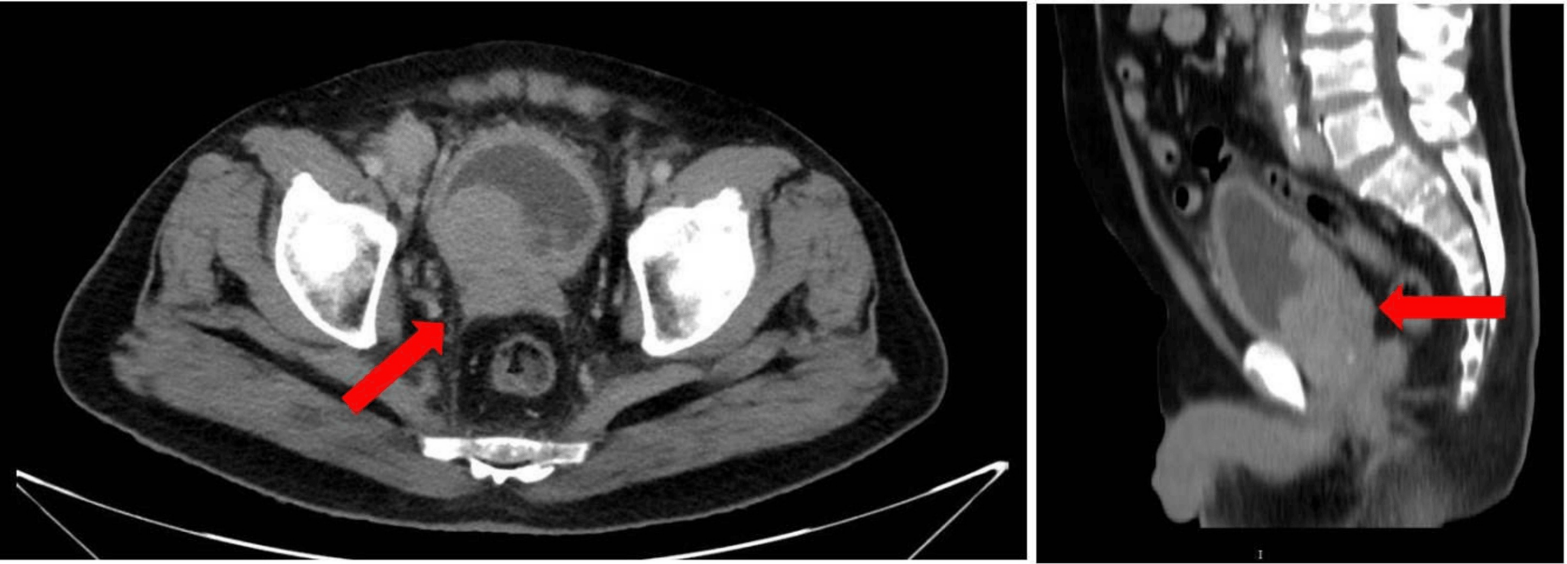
MSCT revealed cystic mass arising from the prostate, causing compression and invasion of the posterior bladder wall (arrows). MSCT: multislice computed tomography
